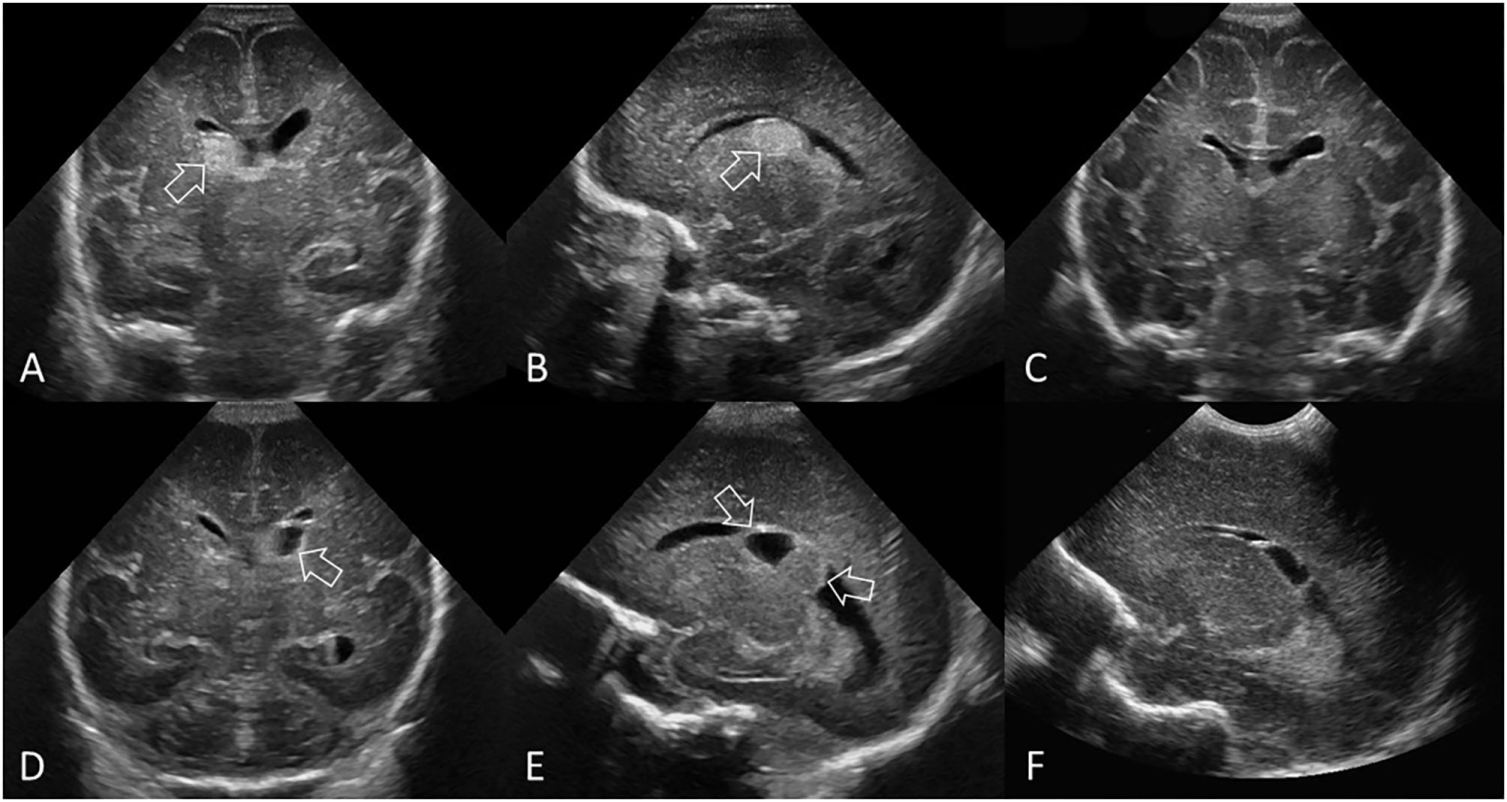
Grade I GMH-IVH. (A–C) Ultrasound scan in a preterm infant (GA 26 weeks) with common pattern of grade I GMH-IVH. (A) Coronal scan at the level of the frontal horns of the lateral ventricles. (B) Parasagittal scan through the right lateral ventricle. The location of the echogenic clot (arrow) at the right caudothalamic notch is typical of grade I GMH-IVH. There is no IVH or ventricular dilatation. (C) Follow up coronal scan 12 weeks later shows complete resolution of the GMH. (D–F) Ultrasound scan in a different preterm infant (GA 27 weeks) with a less common location of grade I GMH-IVH. (D) Coronal scan at the level of the frontal horns of the lateral ventricles. (E) Parasagittal scan through the left lateral ventricle. The clot (arrows) is centered posterior to the left caudothalamic notch, but is still considered a grade I GMH-IVH as there appears to be no blood within the ventricles. The clot is non-uniform in its echogenicity, with the echolucent portion representing cystic degeneration (liquefaction) typical of an “older” GMH-IVH at this stage. There is no ventricular dilatation. (F) Follow-up parasagittal scan 9 weeks later shows further cystic degeneration and smaller clot size.
